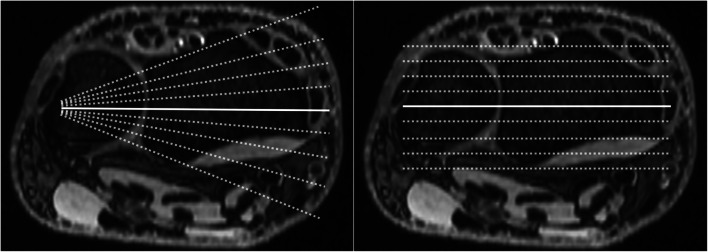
Multiplanar reformatting of isotropic thin-slice MRI in radial (left) and coronal (right) orientation based on axial planes through the distal radioulnar joint
